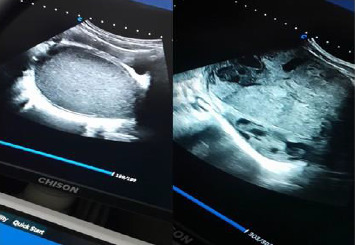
Abdominopelvic ultrasound revealing a large, heterogeneously hypoechoic mass displacing the right ovary.
